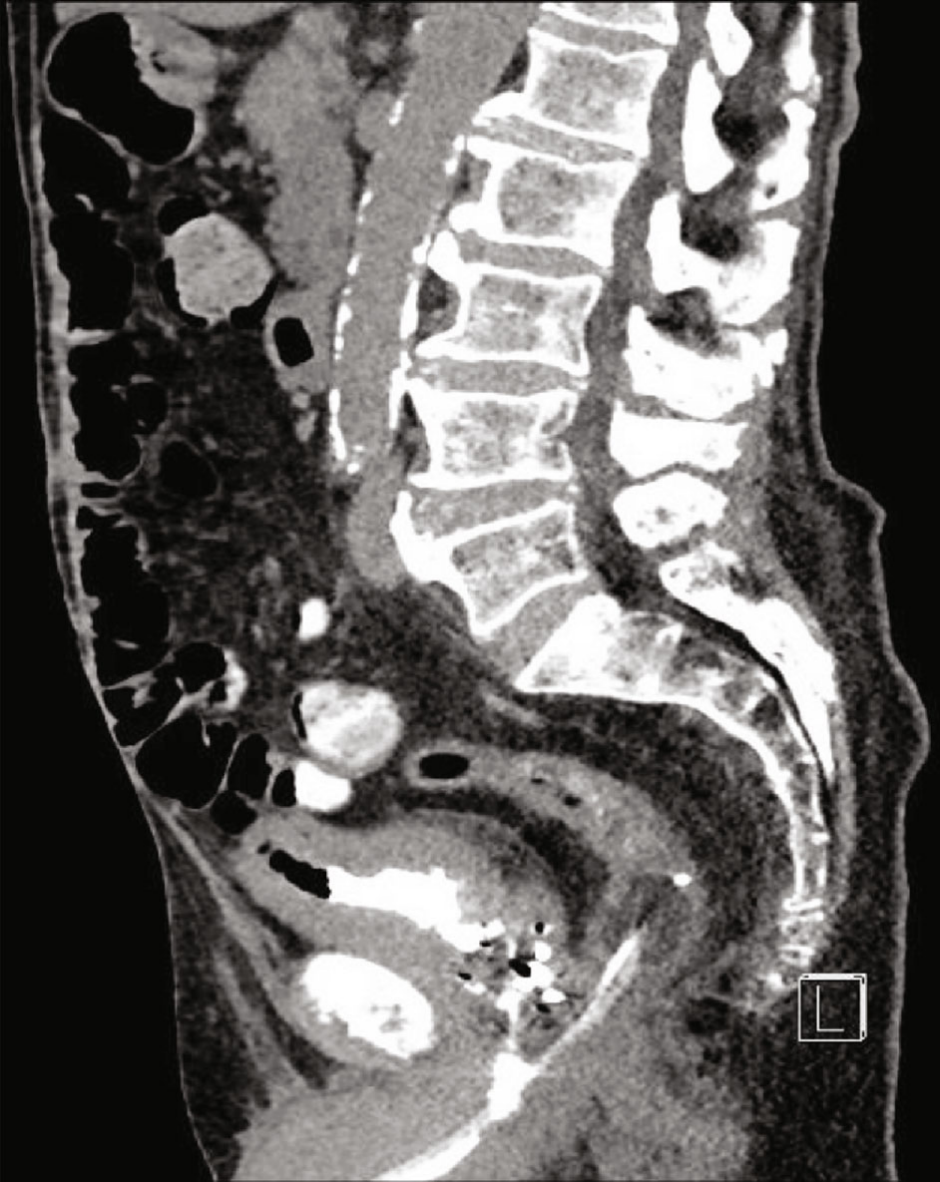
Sagittal CT demonstrating catheter placement in rectum.
